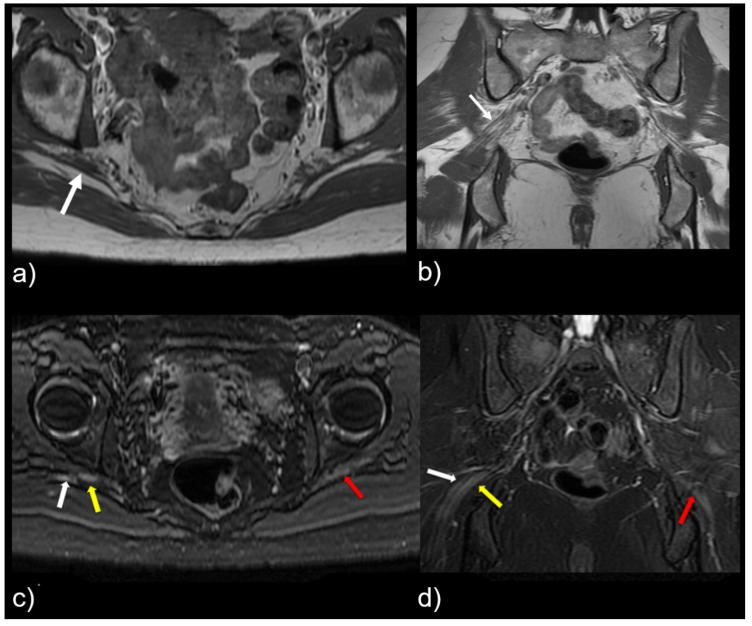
Patient with a symptomatic type II variant and a split right sciatic nerve. Axial (a) coronal (b) T1-weighted sequence, showing the two components of the split sciatic nerve (white arrow). Axial (c) and coronal (d) T2-weighted IDEAL sequences demonstrate the split sciatic nerve, with the common peroneal (white arrow) and the tibial (yellow arrow) nerve components seen distinctively. There is also increased nerve caliber and increased nerve T2 signal involving the split right sciatic nerve, relative to the normal caliber and T2 signal of the left sciatic nerve (red arrow).
